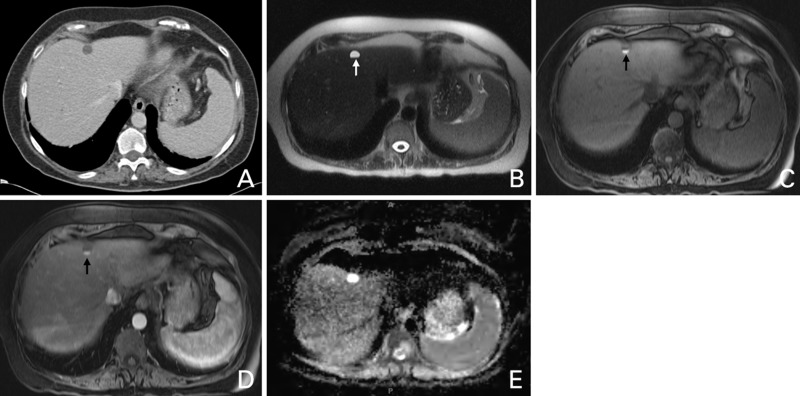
A 62-year-old woman with ciliated hepatic foregut cyst. Contrast-enhanced CT (A) shows a hypodense lesion in segment 4 A, with water attenuation. The lesion is bright on T2 WI, with a dark dependent layer (arrow) (B). The dependent layer is bright on T1 weighted images pre- (C) and on arterial post-contrast (D) images (arrows), suggestive of a fatty or proteinous nature. There is no associated diffusion restriction, as can be seen on the ADC image (E).
